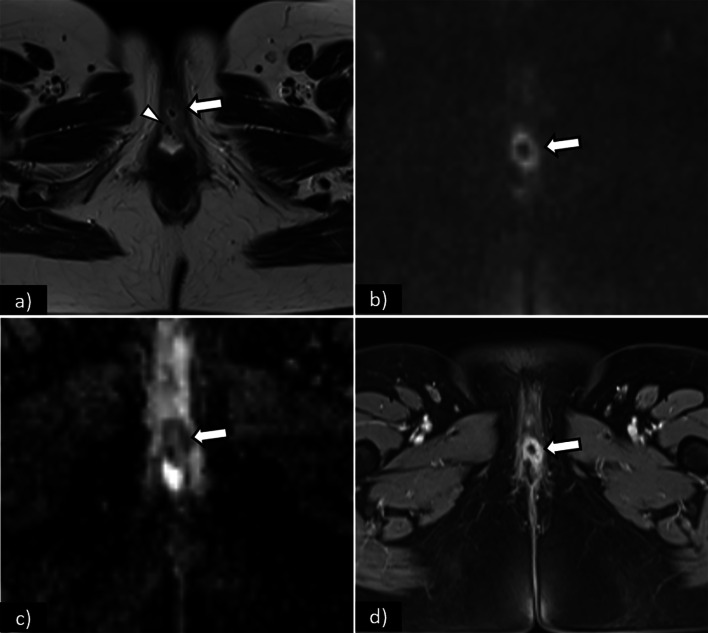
Axial T2WI (a) shows an intermediate signal intensity tumour (arrow), measuring 3 cm, with central necrosis and invasion of the external urethral meatus (arrowhead)—FIGO II. On DWI, the tumour is depicted by a high-signal intensity lesion on DWI (*b*-value = 1000 s/mm^2^) (arrow), and low-signal intensity on the corresponding ADC map (arrow). On T1WI fat saturation contrast-enhanced sequence (d), the tumour shows early arterial enhancement of its solid component (arrow), with no enhancement of the central necrotic portion. Note the increased conspicuity of the lesion in DWI-MRI (b) and c and in DCE-MRI (d)
